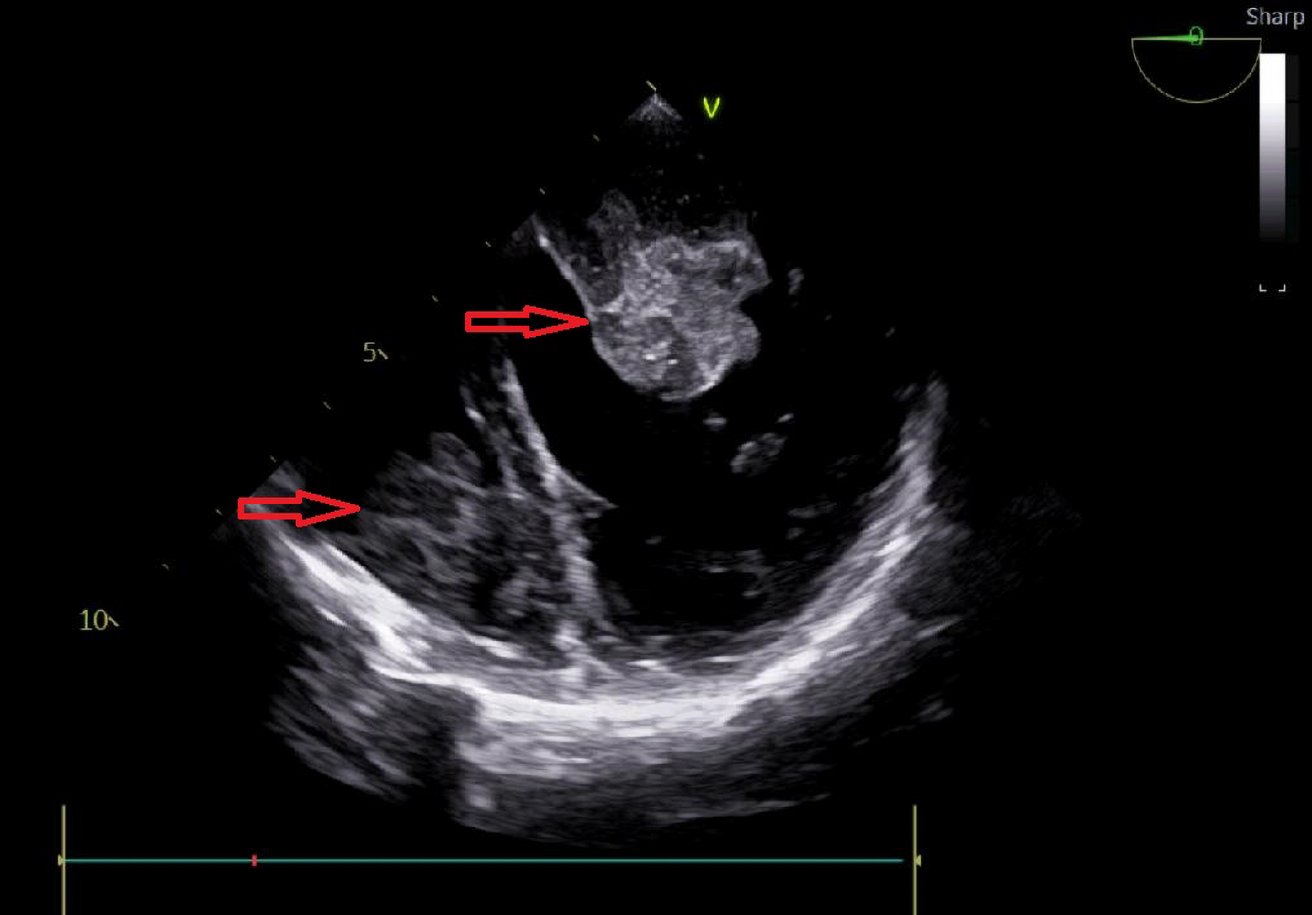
Transesophageal echocardiography, the mass shadow at the arrow is myxoma.
